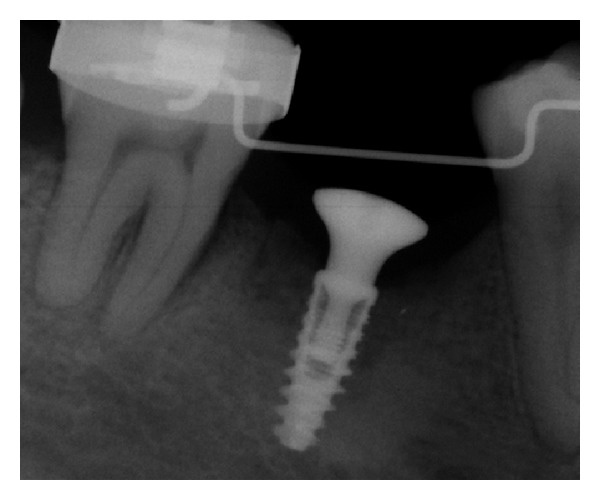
Radiographic control of the healing abutment adjustment.
